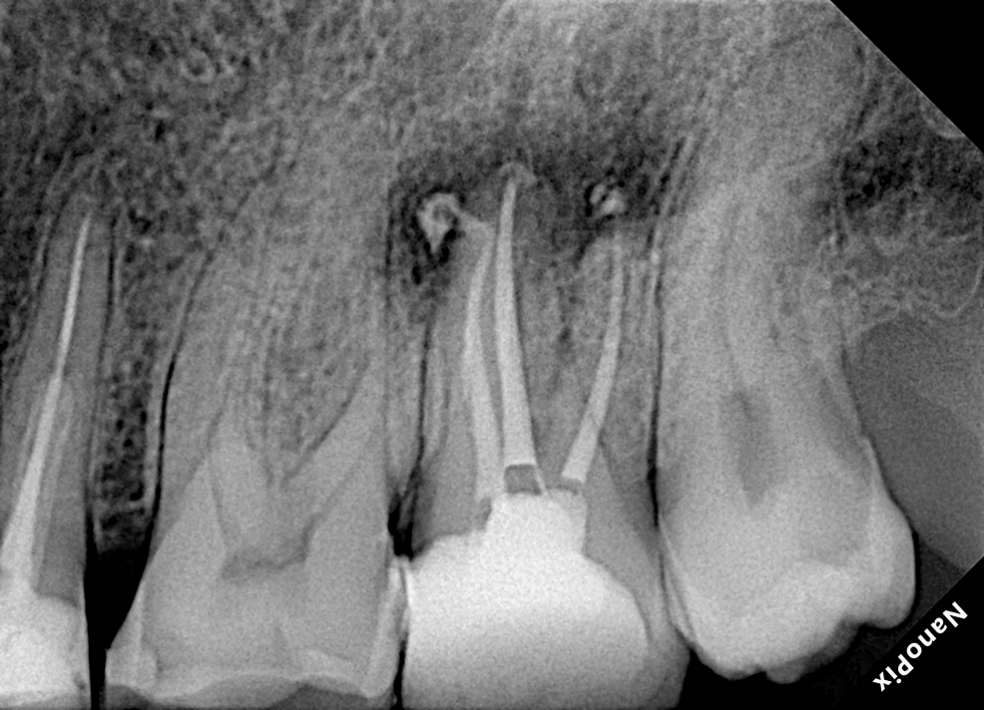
Postoperative periapical radiograph after the completion of root canal filling procedures
